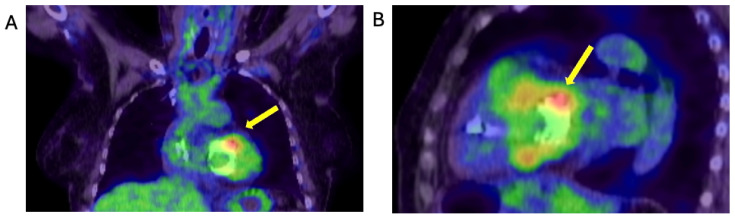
FDG-PET CT after MViV implantation and relapsing enterococcus faecalis bacteraemia. FDG-PET-CT images of chest show focal tracer accumulation over MVR (yellow arrows), which is also present on non-attenuation correction images, suggestive of underlying infective endocarditis. Cardiac pacemaker leads, and generator showed no tracer accumulation. (A): coronal and (B) sagittal PET CT images of chest.
